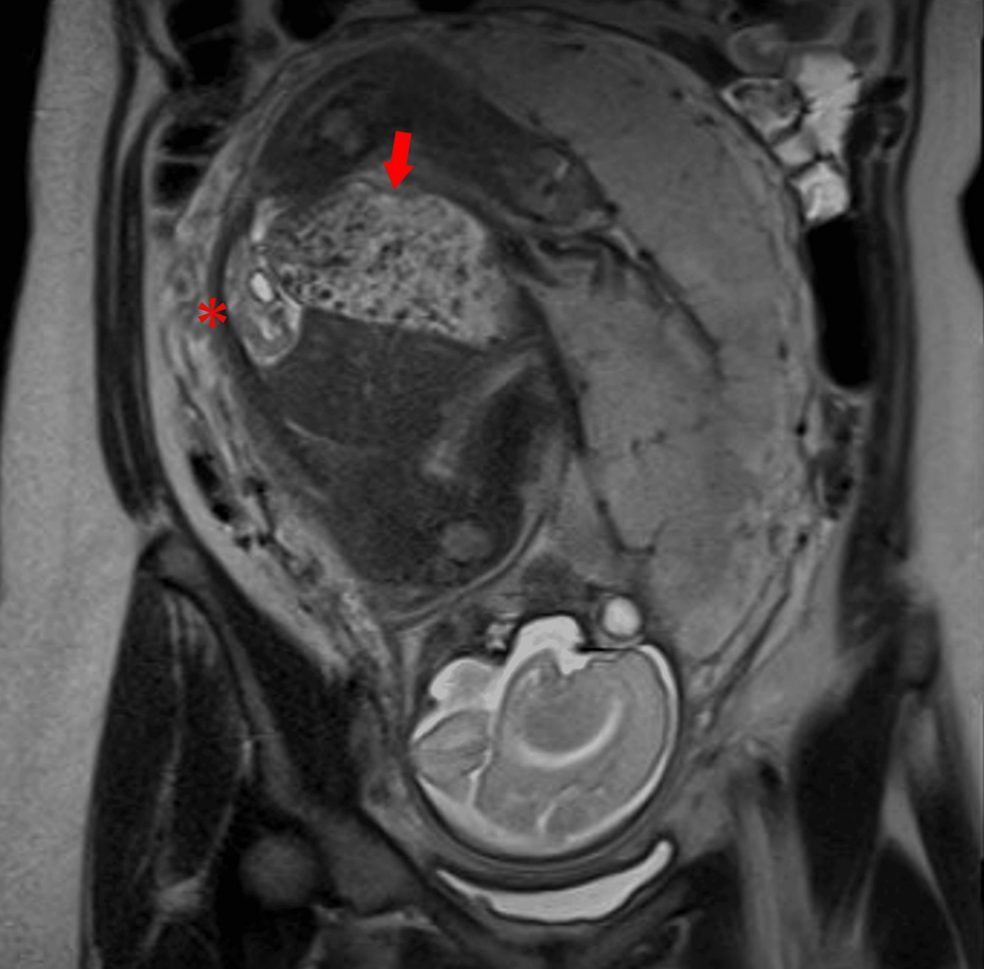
Fetal magnetic resonance imaging at 29 weeks of gestation Fetal magnetic resonance imaging showing anhydramnios and intestinal dilation containing low signal intensity nodules (arrow). Kidney sizes are normal (*).
